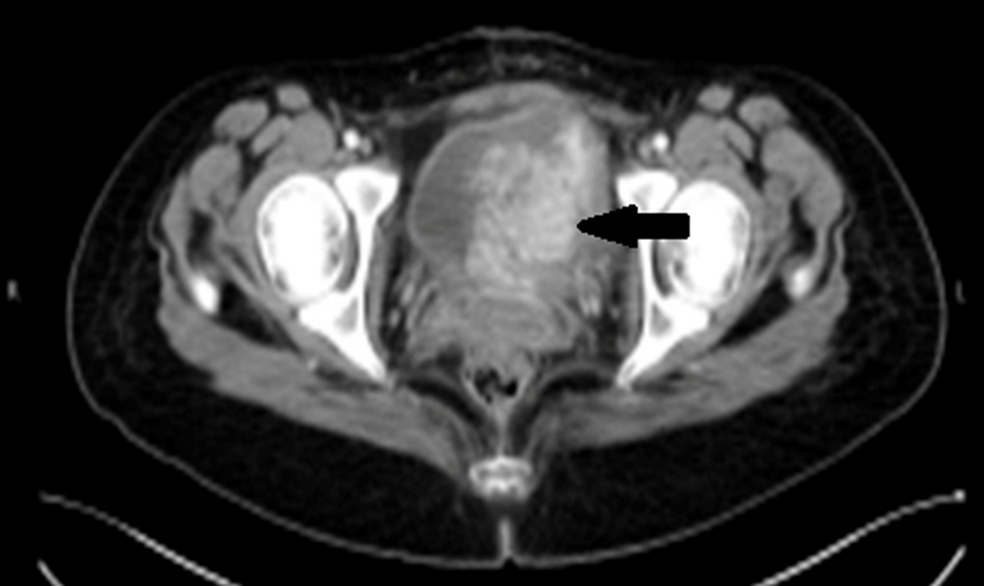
CT of the pelvis (axial section) showing large irregular soft tissue mass arising from the left lateral wall and base of the bladder, measuring 6.1 x 6.0 x 6.0 cm. CT, computed tomography
